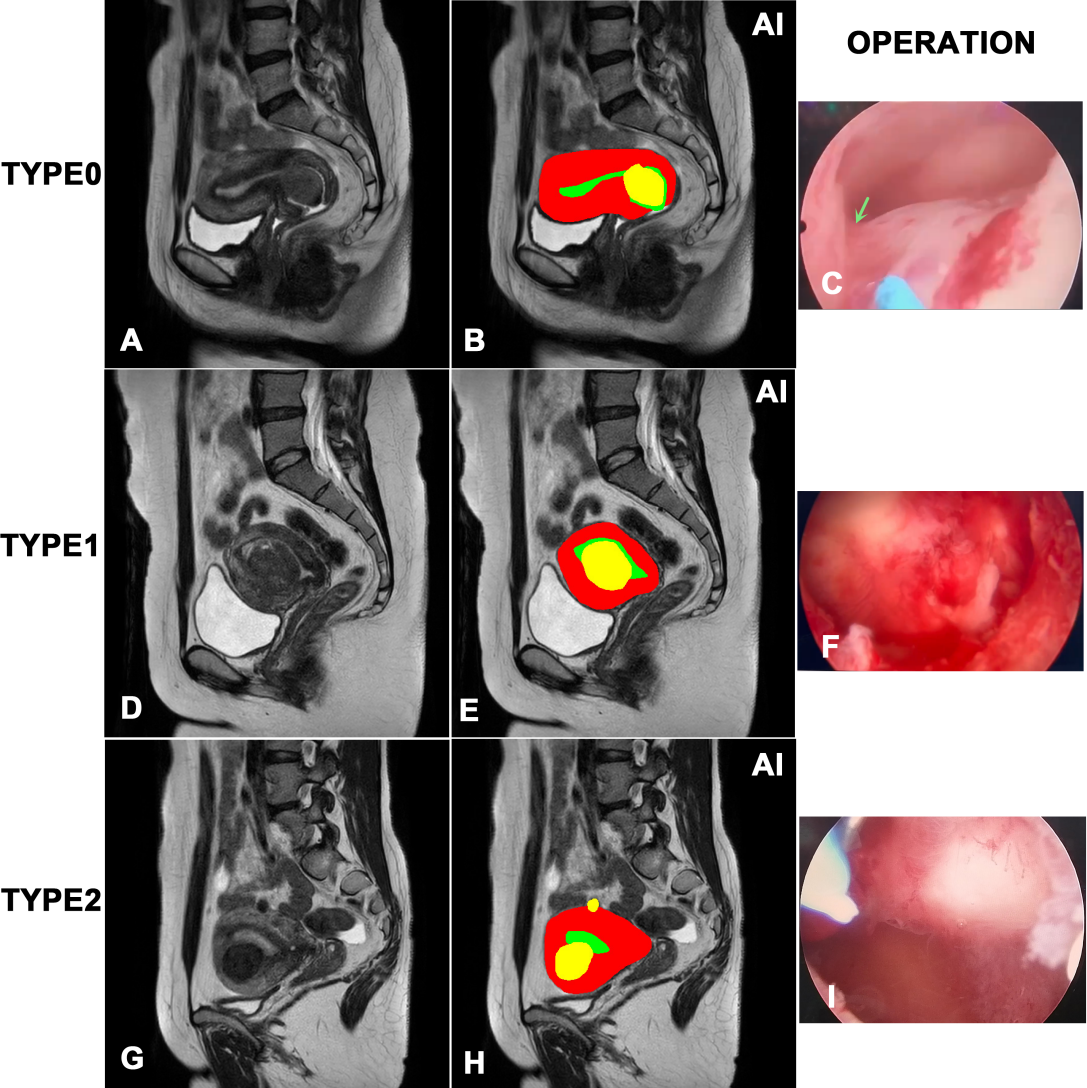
Visualization of the instance segmentation of our AI model. The left side represents original MRI image, and the right side represents the inference masks generated by our AI model. In the masks of model inference, yellow represents myomas, red represents the uterine wall, green represents the uterine cavity. (A, B) and (C) represents type 0 myoma. (D, E) and (F) represents type 1 myoma. (G, H) and (I) represents type 2 myoma.
